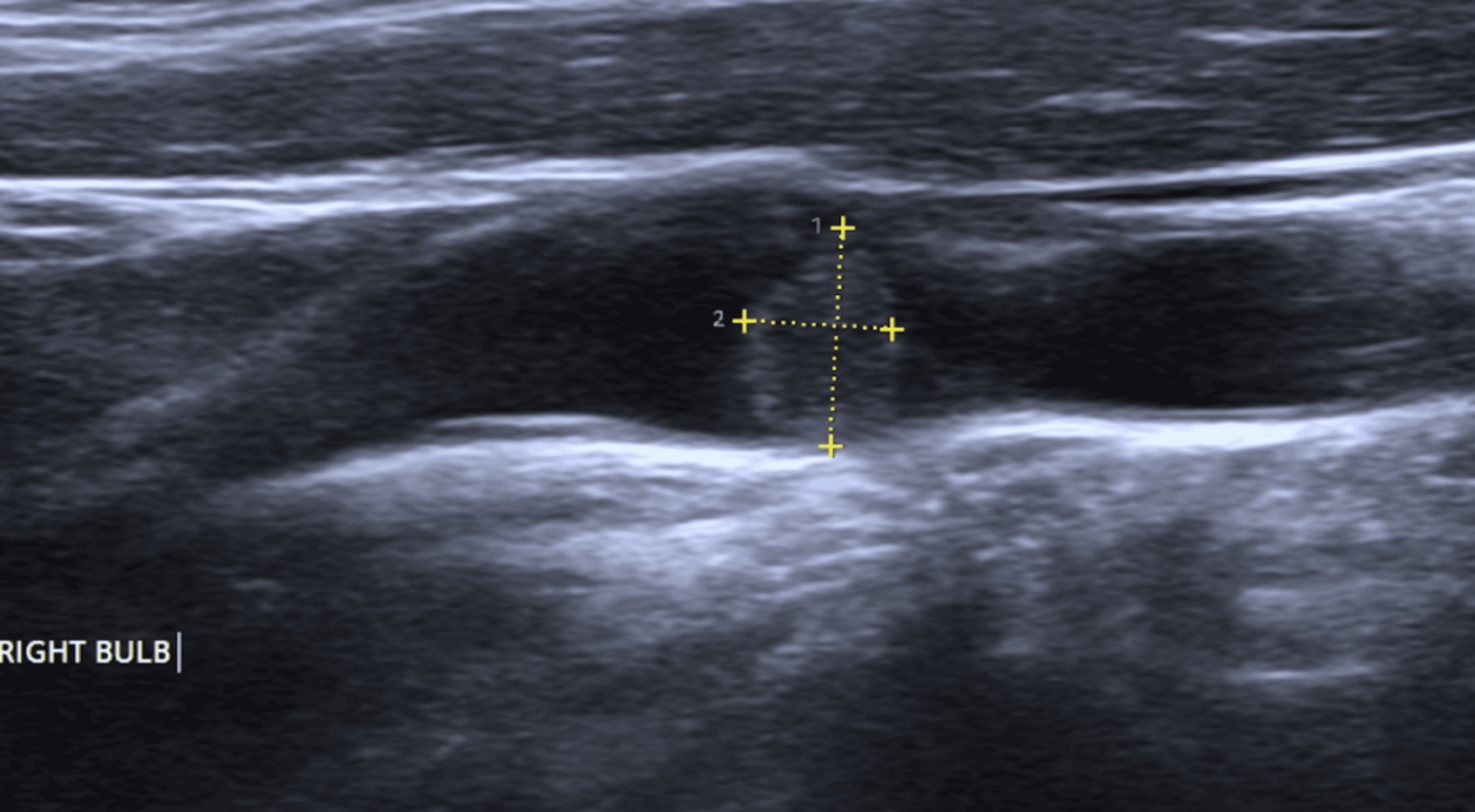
Carotid artery ultrasound Showing a soft plaque measuring 6.4 mm x 4.3 mm in the right carotid bulb.
